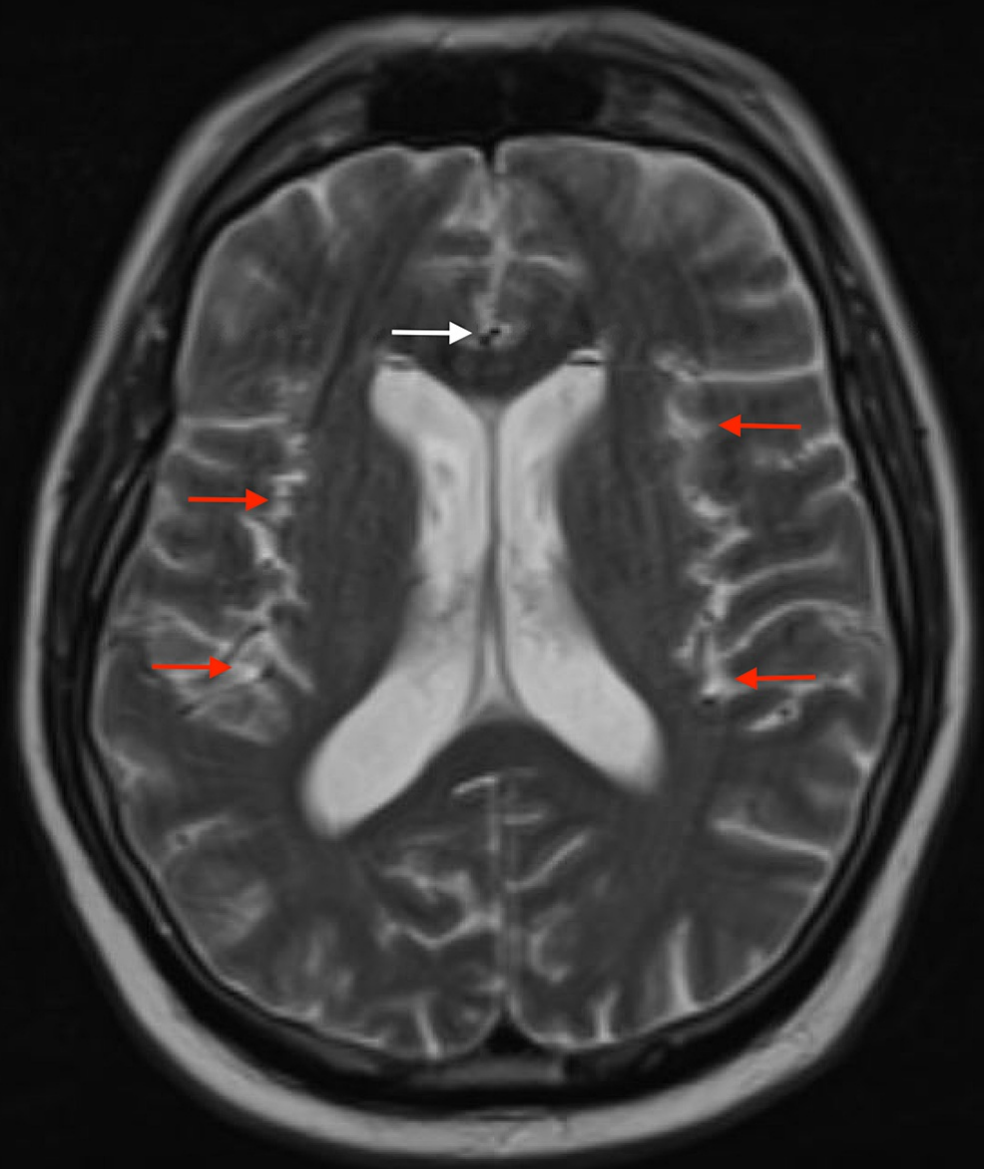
Brain MRI T2 axial view Brain MRI axial T2: hyperintensity signals with heterogeneous appearance; Red arrows show hyperintensity signals: fat drops; White arrow shows hypodense signals at frontal area and represent fluid signal.
